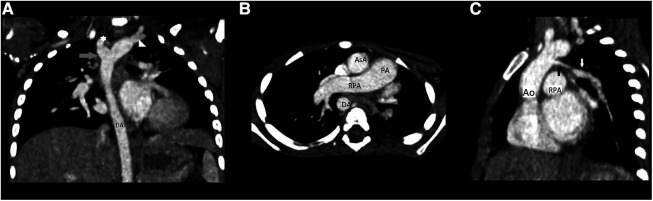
(A) Chest CT angiography scan, coronary reconstruction: elongation of the aorta and an aberrant right subclavian artery with KD; (B) chest CT angiography scan: absence of the left pulmonary artery; (C) chest CT angiography reconstruction: atypical vascular branches dividing from the aorta and forming the vasculature of the left lung; Abb.: Ao, aorta; AsA, ascending aorta; DA, descending aorta; PA, pulmonary artery; RPA, right pulmonary artery; star, abberant right subclavian; triangle, left subclavian artery; grey arrow, KD; black arrow, atypical branch; white arrow, anomalous left lung vascular bundle.
